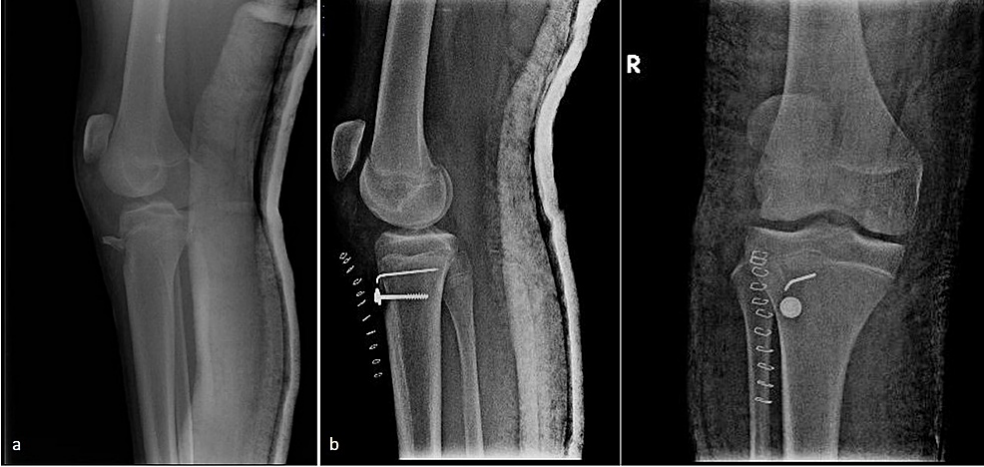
Initial radiographs. (a) Preoperative lateral radiography of the patient’s right knee showing a displaced tibial tubercle fracture (Ogden type IB); (b) postoperative lateral and anteroposterior radiographs after open reduction and internal fixation of the tuberosity with a cortical screw and one additional KW. This fixation was performed in another hospital and we did not have any information regarding the status of the patellar tendon at that time. KW: Kirschner wire
